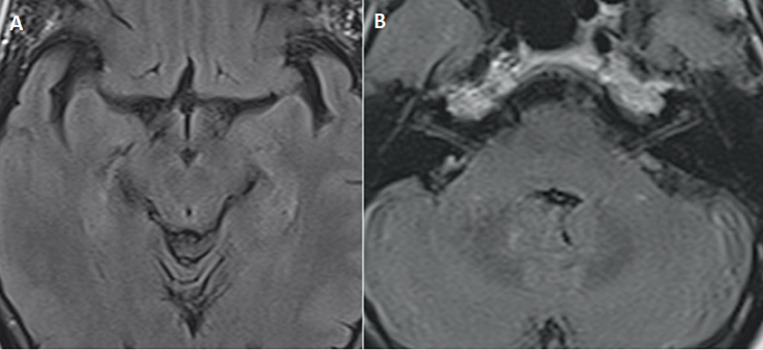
(IRM de contrôle après 4 mois) séquence axiale FLAIR: disparition totale des hypersignaux au niveau périacqueducal, des colliculi et des noyaux dorsaux du pont
